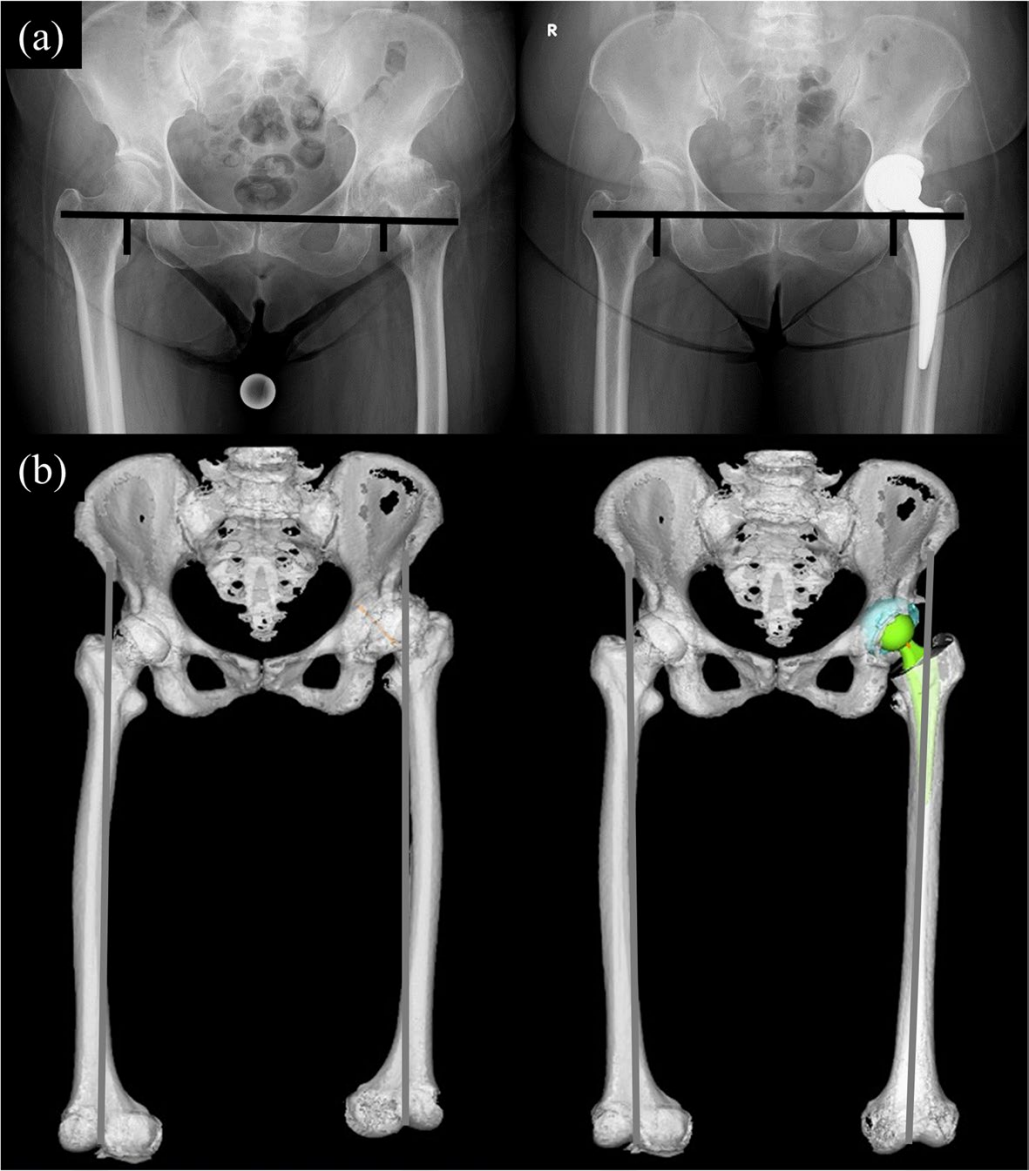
a Assessment of leg length discrepancy on the anteroposterior radiograph. Distance between the line connecting both tear drops and the medial apex of the lesser trochanter was measured. b Assessment of leg length discrepancy on the functional pelvic plane after repositioning using the 3D-Template system. Distance from the anterior superior iliac supine to the intercondylar fossa of femur was measured
